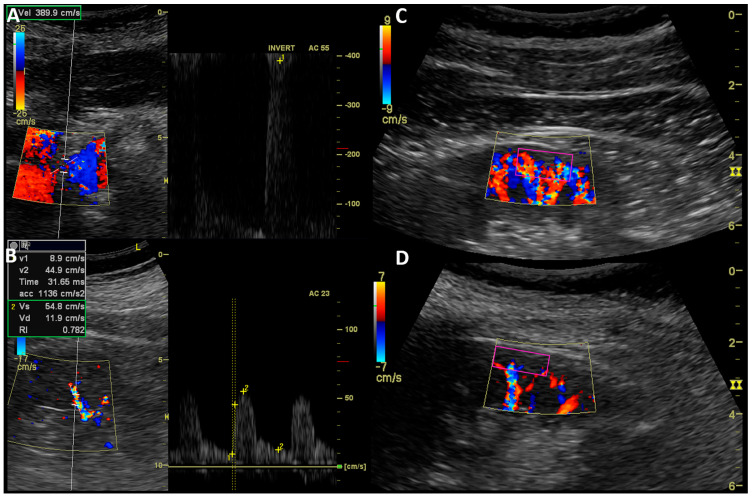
Ultrasound measurement of RAS and kidney perfusion. Doppler ultrasound examination: (A)—Measurement of peak systolic velocity in stenosis of right renal artery; (B)—Acceleration, acceleration time, and resistive index assessment in interlobar artery of left kidney; (C)—cortical perfusion assessment in ROI (purple rectangle) of kidney with insignificant RAS (CSAR 25%, MeD 6.5 mm); (D)—cortical perfusion assessment in ROI (purple rectangle) of kidney with significant RAS (CSAR 80%, MeD 3.5 mm).
